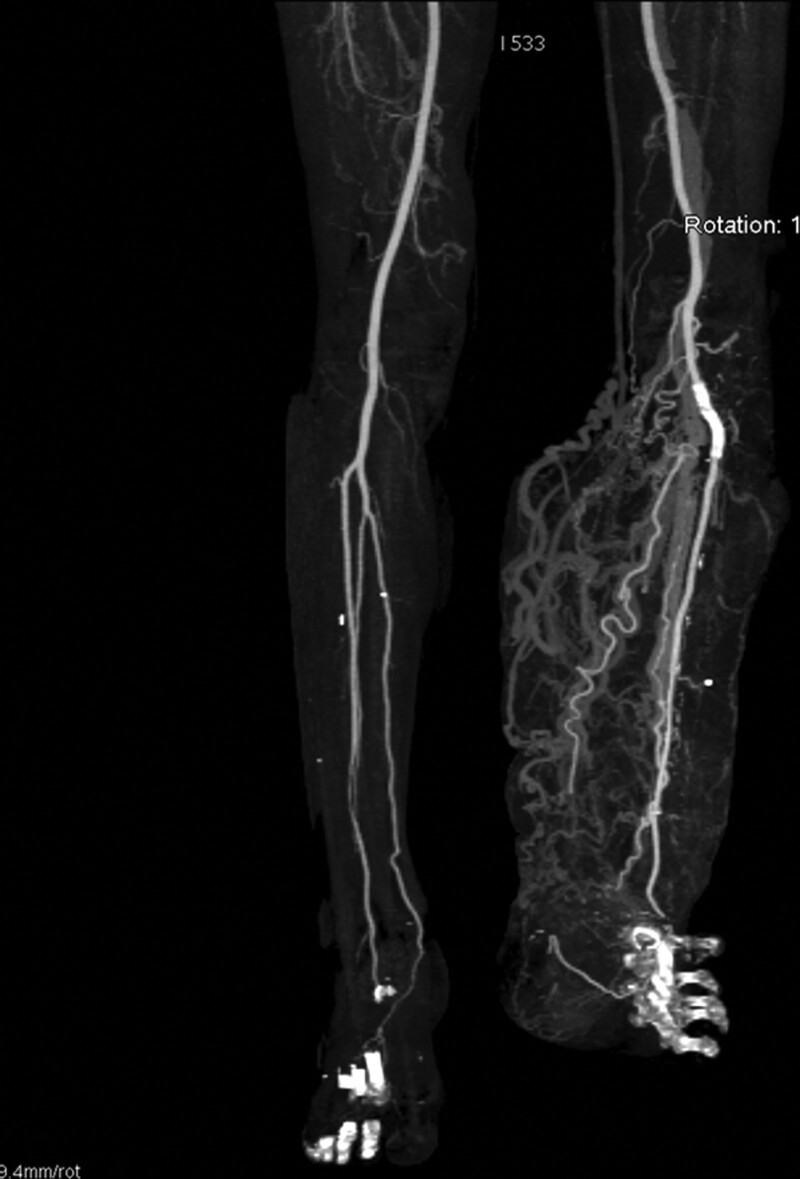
FU CT performed 6 months later showed patent ATA flow through a stent, and the aneurysm had disappeared. ATA = anterior tibial artery, CT = computed tomography.
